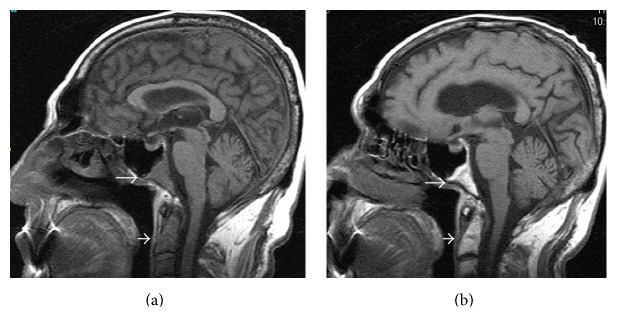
Initial sagittal nonenhanced T1-weighted MRI (a) show hypointensity of the clivus (arrow) and cervical vertebrae (short arrow) corresponding to infiltration. Eight months later after systemic therapy sagittal nonenhanced T1-weighted MRI (b) shows significant reduction of the hypointensity of the both clivus (arrow) and cervical vertebrae (short arrow).
